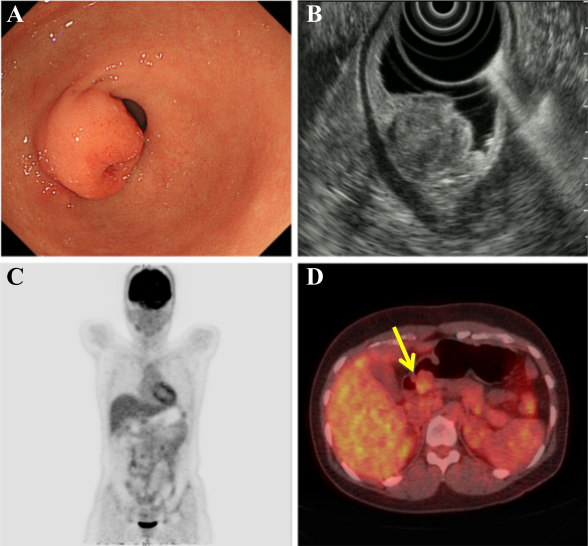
a Upper gastrointestinal endoscopy revealed an elevated mucosal lesion in the pyloro-antral region with erosive changes. b Endoscopic ultrasonography revealed a heterogeneous tumor measuring 20 mm that was not connected to the muscularis propria of the stomach. c, d A positron emission tomography/computed tomography scan did not show significant uptake of ^18^F-fluorodeoxyglucose into the tumor (*arrow*)
